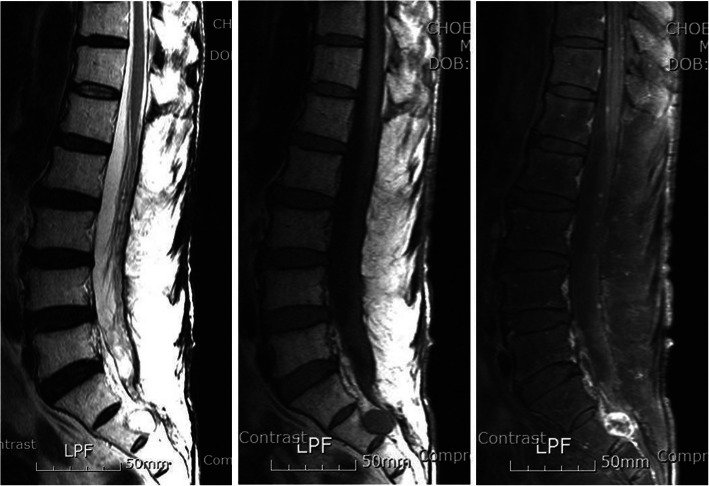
Magnetic resonance imaging at postoperative 2-year follow-up shows almost complete removal of the large mass at the lumbar spine, as well as three round small masses at the lumbar spine and a multi-lobulated round masses at S2-S3 with no changes
